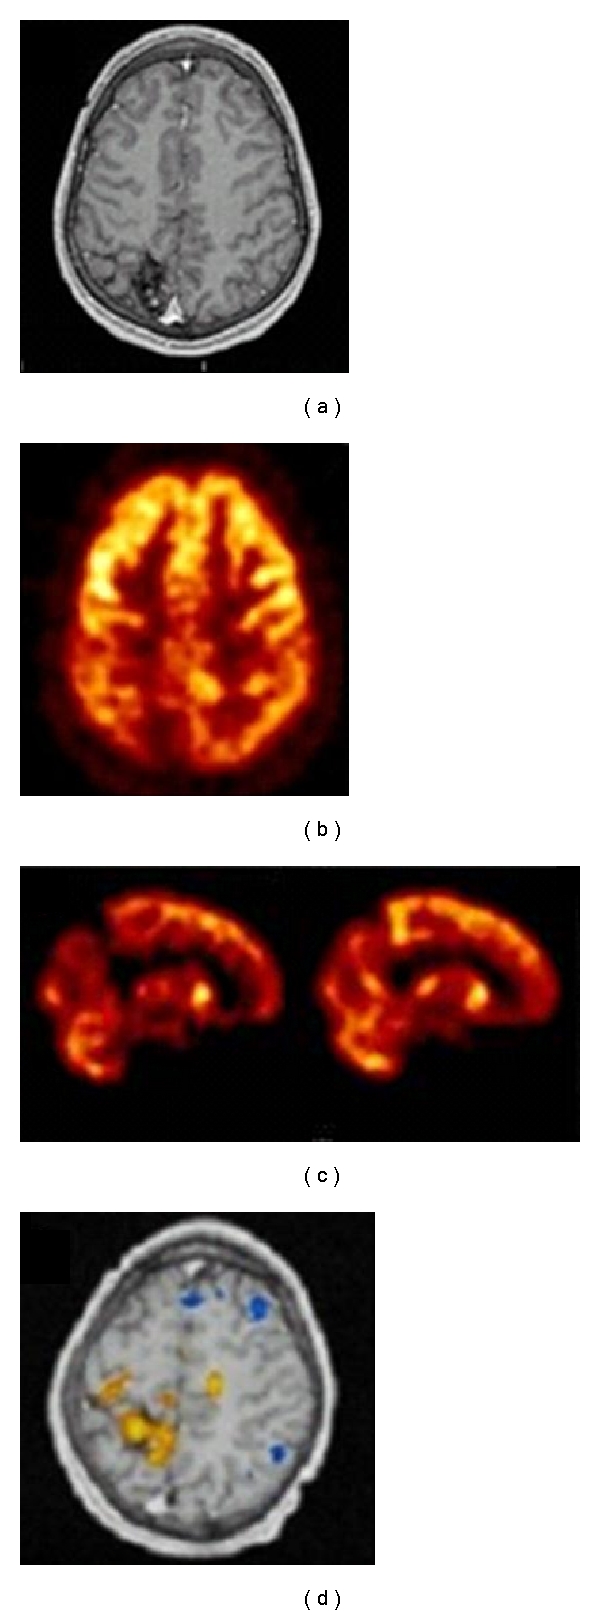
Eleven-year-old male suffering from generalized tonic-clonic seizure disorder. (a) MRI scan of an-11-year old boy suffering from seizure disorder since the age of 7. The patient has tonic-clonic seizures. The MRI scan shows an arterial venous malformation in the right parietal lobe. (b) The interictal brain F-18 FDG PET scan shows an area of reduced metabolic activity in the right parietal lobe consistent with the location of the arterial venous malformation. (c) Images from an interictal Tc-99m ECD brain SPECT (top) as compared to an ictal technetium-99m ECD brain SPECT scan (bottom). One can see significant hyperemia anterior and inferior to the region of the arterial venous malformation on the ictal Tc-99m ECD brain SPECT scan as compared to the interictal brain SPECT scan. (d) SISCOM analysis where the ictal and interictal SPECT are compared and statistically significant differences between the two are mapped onto the patient's MRI scan. One can see significantly increased differences in the ictal study as compared to the interictal study in the anterior region and in the location of the arterial venous malformation (highlighted yellow areas). The highlighted blue area shows areas of decreased uptake on the ictal scan as compared to the interictal scan, which can generally be seen positioned randomly throughout the cortex and do not have clinical or localizing significance.
